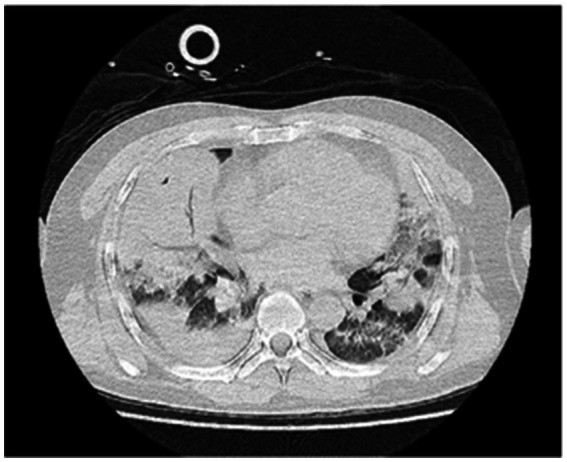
Lung CT scan upon admission.
